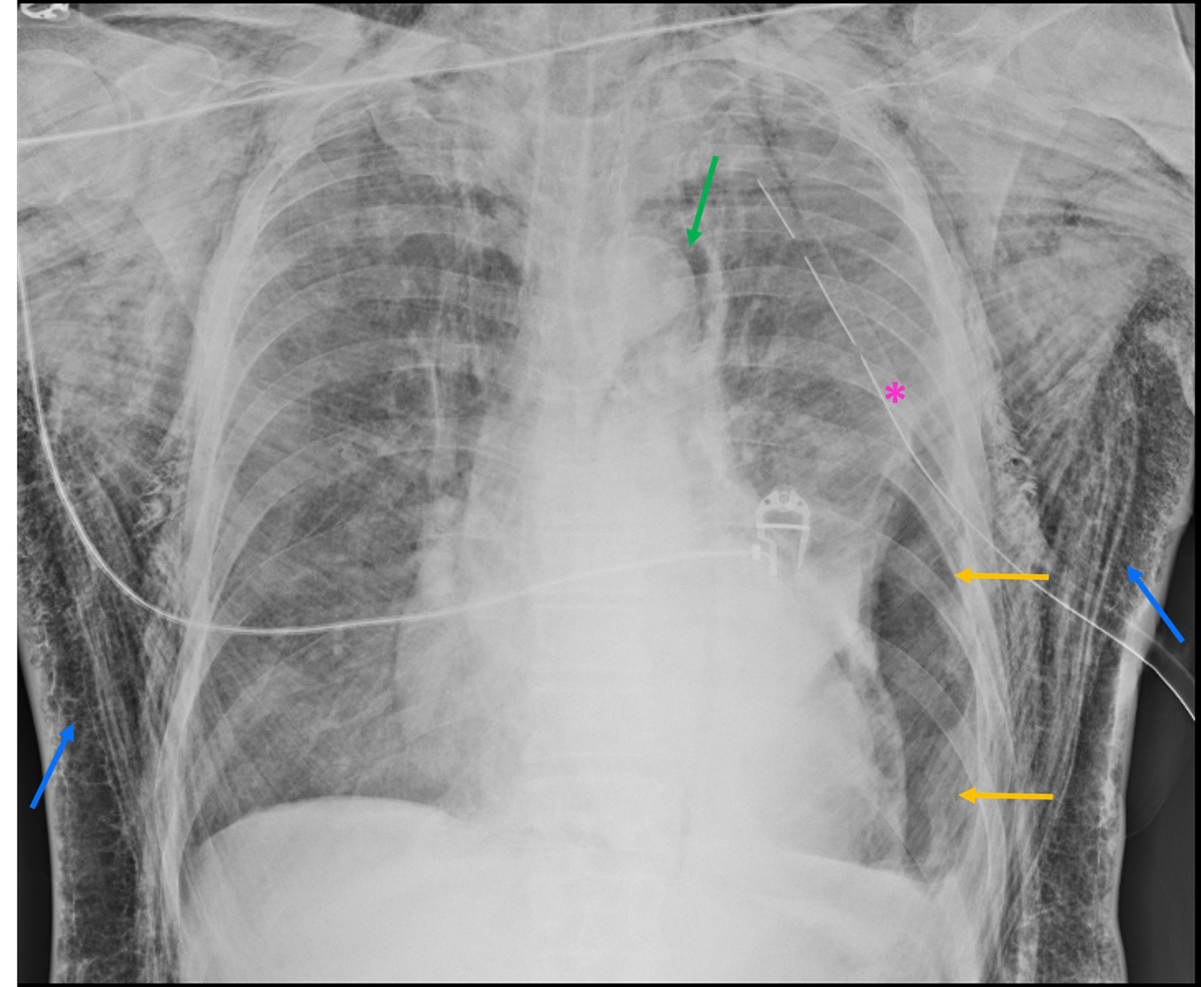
Supine chest radiograph Supine chest radiograph that shows a left basal pneumothorax (yellow arrows), pneumomediastinum (green arrow) and extensive thoracic subcutaneous emphysema (blue arrows). Chest tube (pink asterisk).
